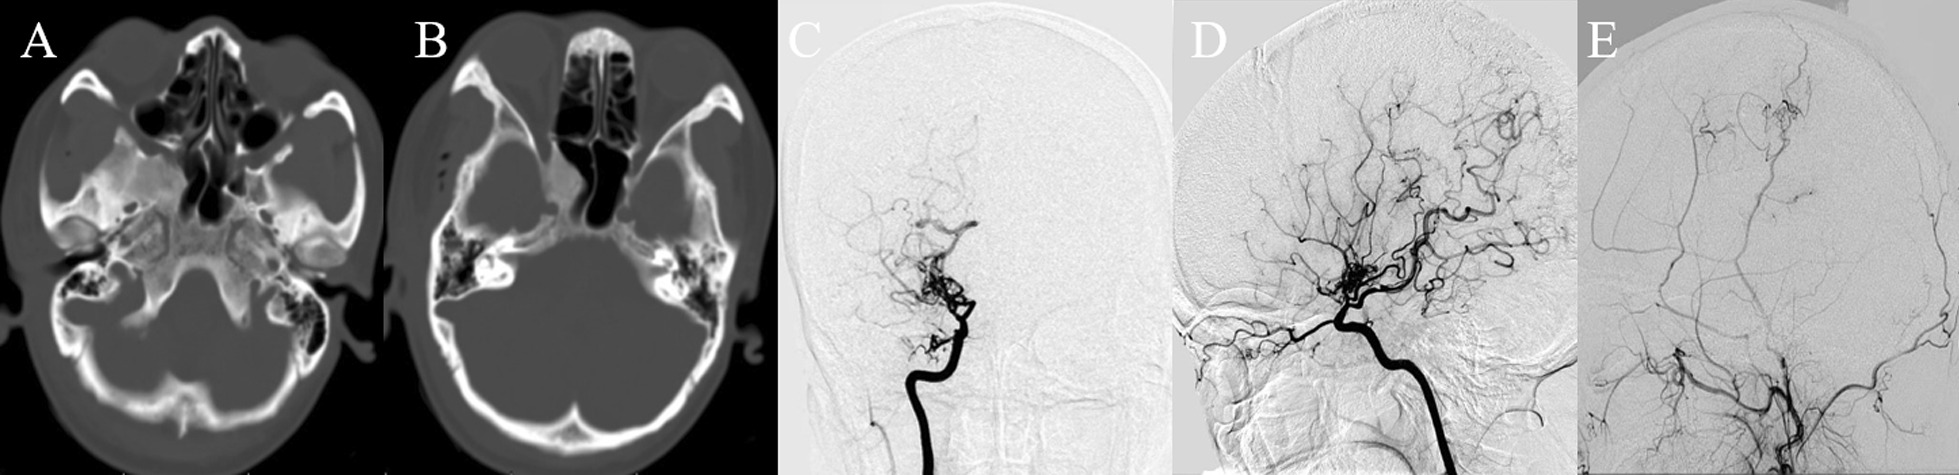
CT showing FD of local right sphenoid bone (A, B) and DSA showing MMD with the terminal of right internal carotid artery occluded (C, D). Right indirect revascularization was done and after 3 years, the DSA showed neovascularization (E)
